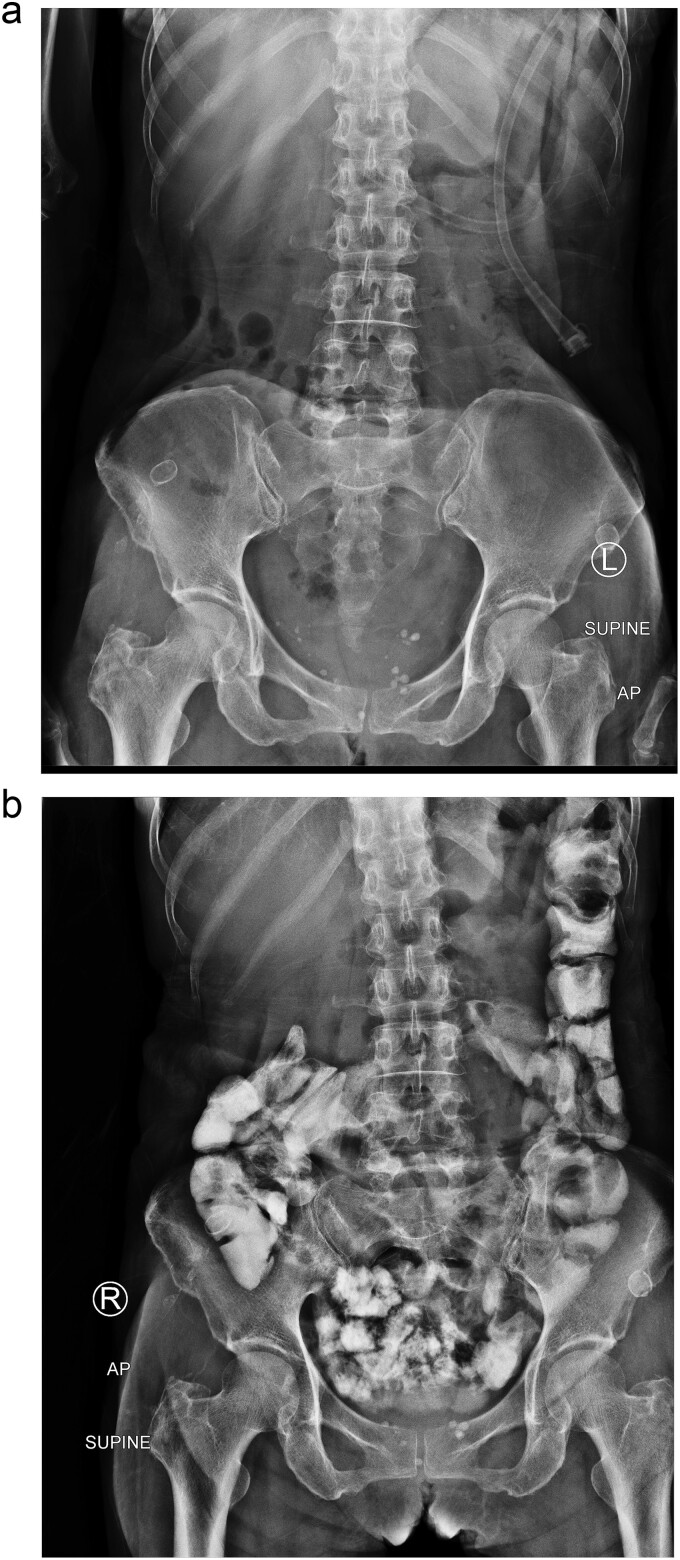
Two weeks of conservative treatment: (a) abdominal plain film, (b) oral contrast study.
